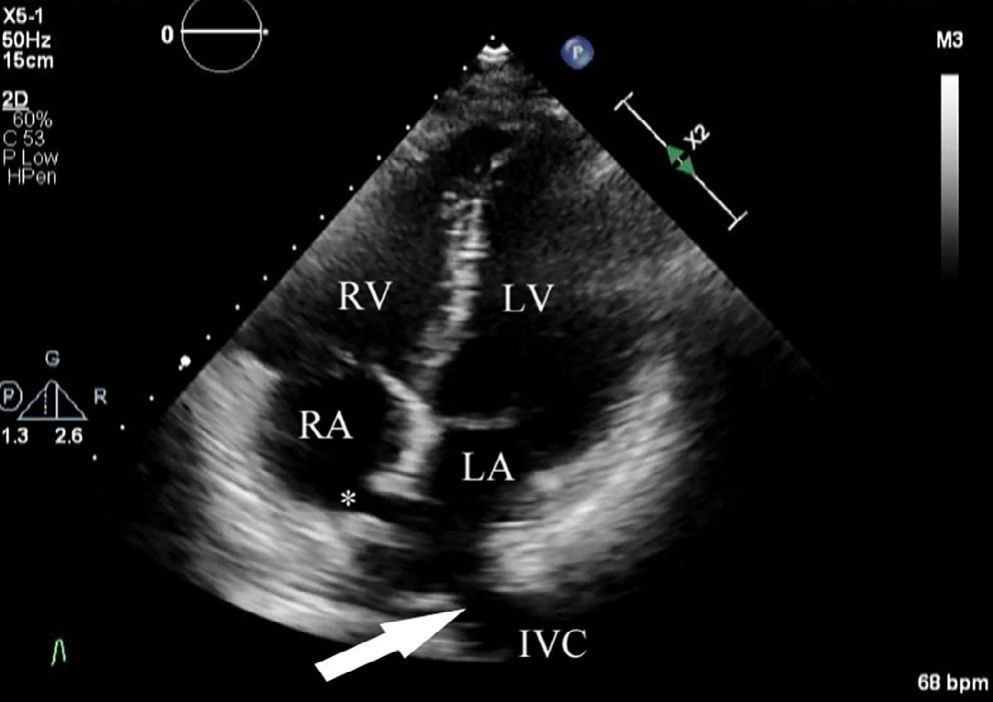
Preoperative transthoracic echocardiography image: The apical 4‐chamber view demonstrates residual atrial septal defects (asterisk) with the inferior vena cava directed to the left atrium (arrow). IVC, inferior vena cava; LA, left atrium; LV, left ventricle; RA, right atrium; RV, right ventricle
